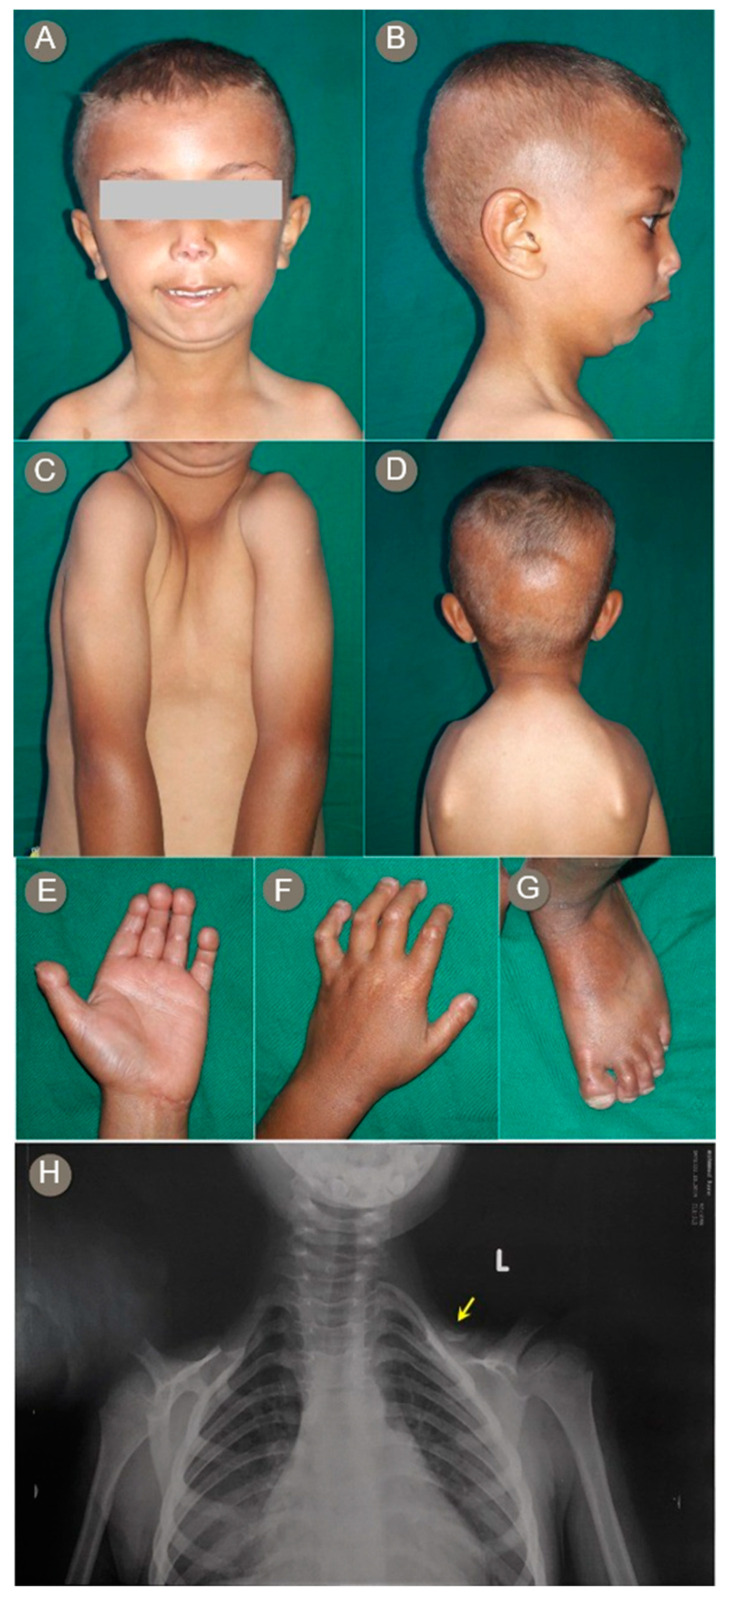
Proband 2 showing an oval face; wide eyes with periocular hyperpigmentation; arched eyebrows; a short, thin, upturned nose; a small mouth with limited opening (A); submental obesity and microretrognathia (B); narrow, sloping shoulders and prominent scapular wings (C); abnormal facility in opposing shoulders (D); short fingers with drumstick-shaped distal phalanges (E); flexed fingers with atrophic shiny overlying skin; hypopigmentation over the knuckles and broad, short, dystrophic nails (F); and round toe tips, nail dystrophy, and visible veins (G). Chest radiograph demonstrating severely hypoplastic/absent clavicles bilaterally (H).
